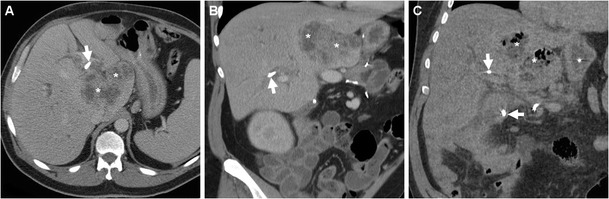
Liver abscess in a 55-year-old man following PD performed at another hospital and complicated by sepsis. CT (a, b) showed development of a multilocular mass with peripheral and septal enhancement (*) in the caudate lobe. Before the patient passed away, repeated unenhanced CT 72 h later (c) showed development of gas in the liver abscess (*). Note external biliary drainage (*thick arrows*)
